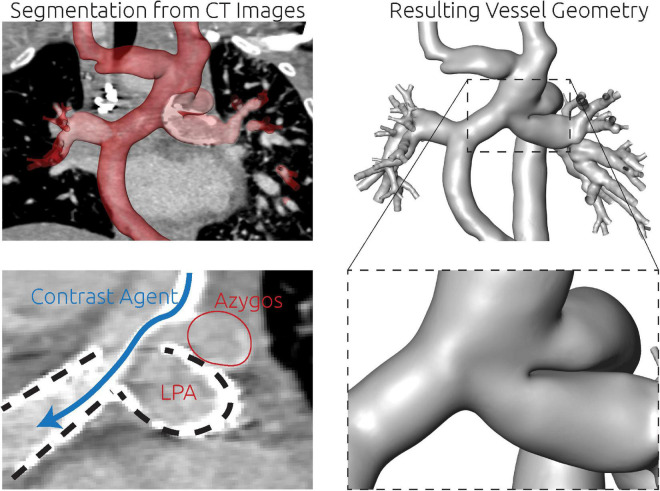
Detailed overview of the segmentation strategy for the third patient. The whole anatomy was reconstructed from CT images (top row). There were two metallic stents in the LPA and RPA (bottom left, dashed lines). Furthermore, the contrast agent deriving from the SVC was unevenly distributed and visible as a high contrasted band flowing toward the RPA (blue arrow). Directly superior to this complex region, also the azygos vein connected to the SVC. The separation between LPA and azygos vein could only be discriminated by the location and orientation of the LPA stent.
